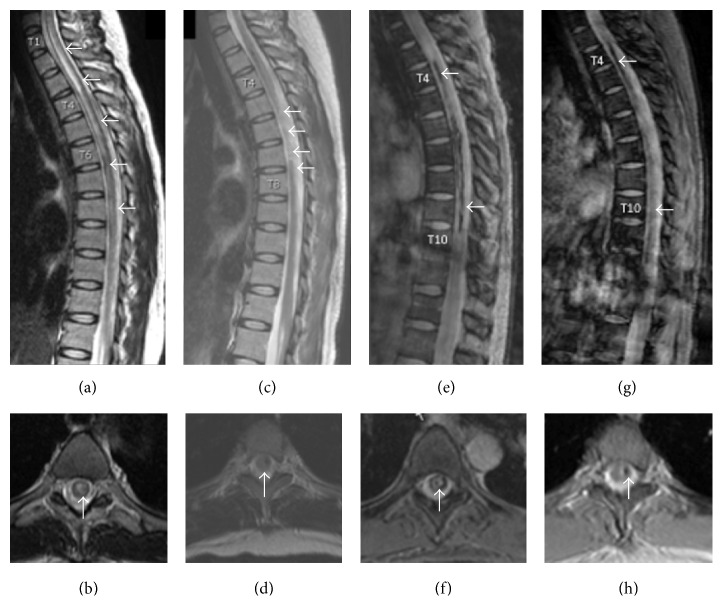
Cervical and thoracic MRI. At initial presentation, sagittal T2-weighted image (a) demonstrated extensive T2-signal abnormality from C6-C7 to T11-T12 involving the central gray matter (b). Sagittal gradient echo (GRE) showed decreased GRE signal extending from T3-T4 to T9-T10 (e, f). At three-month follow-up, the cord signal on T2-weighted imaging was normal (d) with volume loss within the cord from T3-T4 to T7-T8 (c, arrows). The long regions of decreased GRE signal within the central aspect of the cord compatible with hemosiderin deposition persisted (g, h). There was poorly defined abnormal enhancement within the cord behind the T5 and T6 vertebral bodies (not shown).
